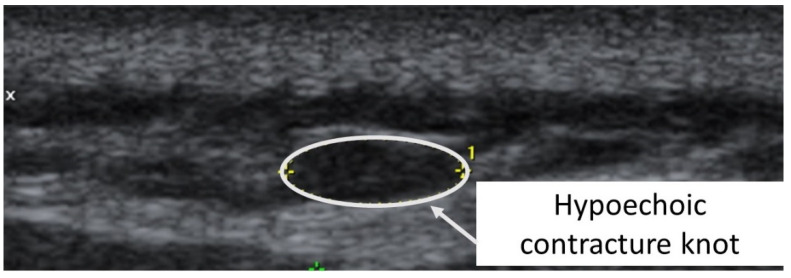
Ultrasound imaging assessment of the left upper trapezius muscle showing a palpable contracture knot as a hypoechoic (hyperperfused) area.
